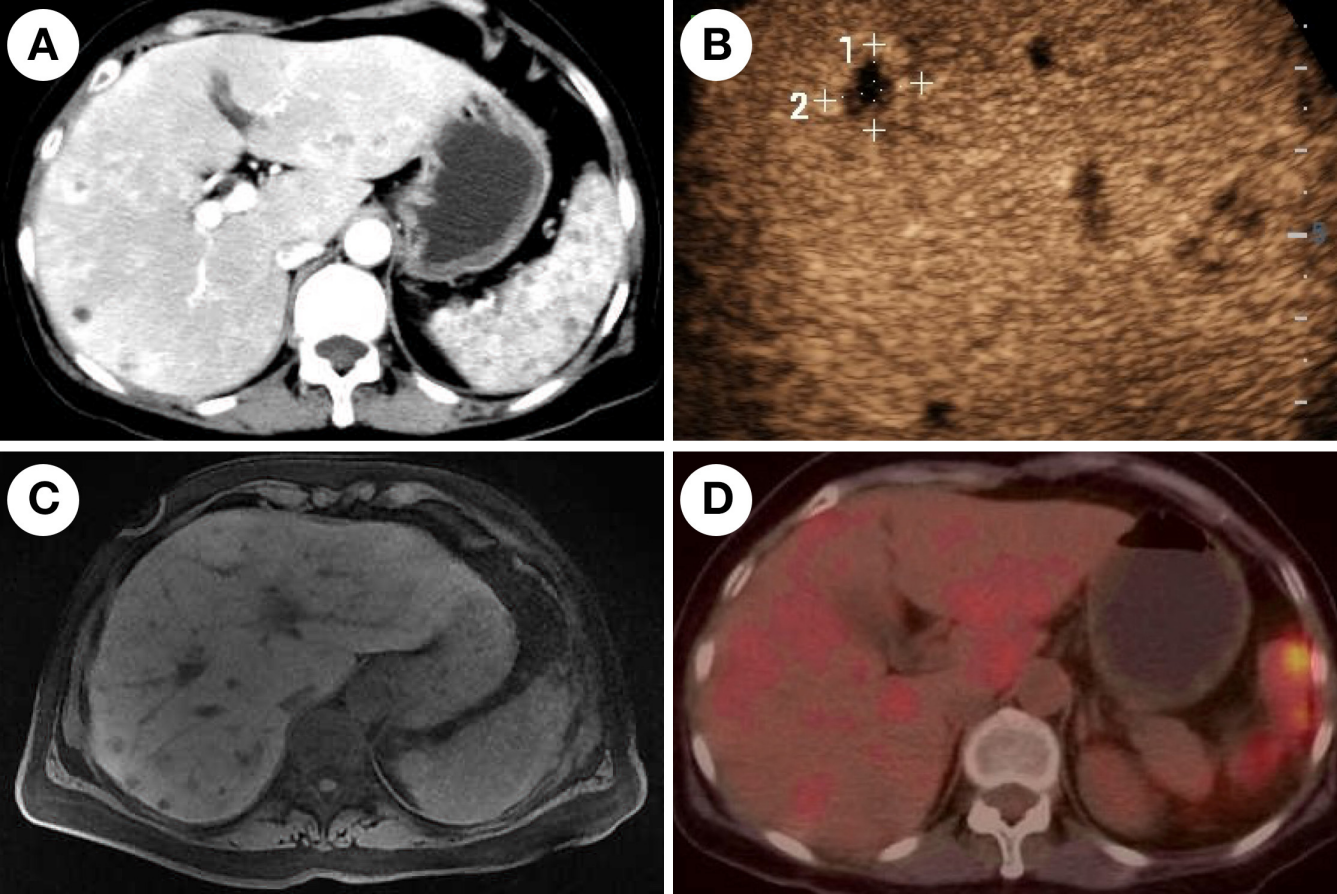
Imaging features of chronic disseminated candidiasis. (A) Contrast-enhanced computed tomography of the abdomen shows multiple nodules with marked rim-enhancement. (B) Contrast-enhanced ultrasound shows multiple hypo-echoic lesions in the liver. (C) Magnetic resonance imaging shows multiple-sized circular abnormal signal lesions in the liver and spleen. (D)
